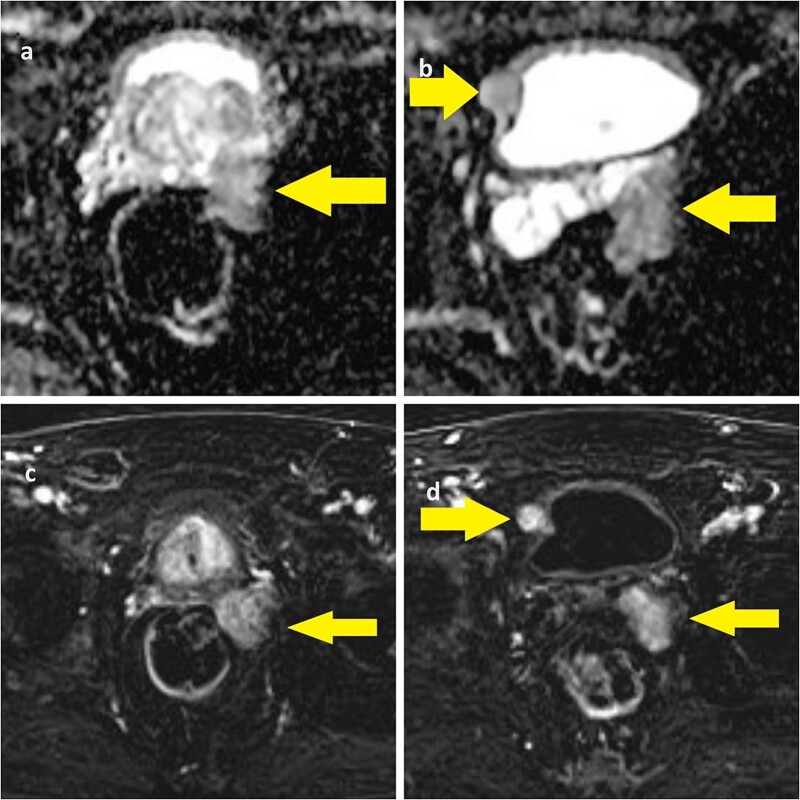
(a) ADC-weighted MRI showing left prostate lobe and left seminal vesicle lesion; (b) T1-weighted MRI showing left seminal vesicle and right paravesical lesion; (c, d) T1-weighted MRI with same lesions.
